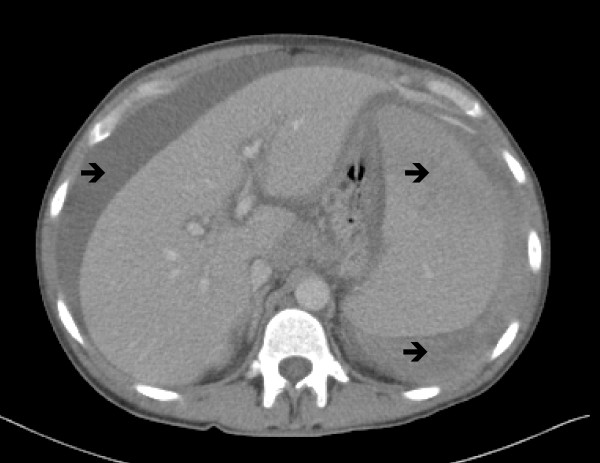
Abdominal computed tomography (CT) showing significant hemoperitoneum, with extravasation of contrast into the right flank/para-colic gutter. Hepatomegaly and splenomegaly are clearly seen.
